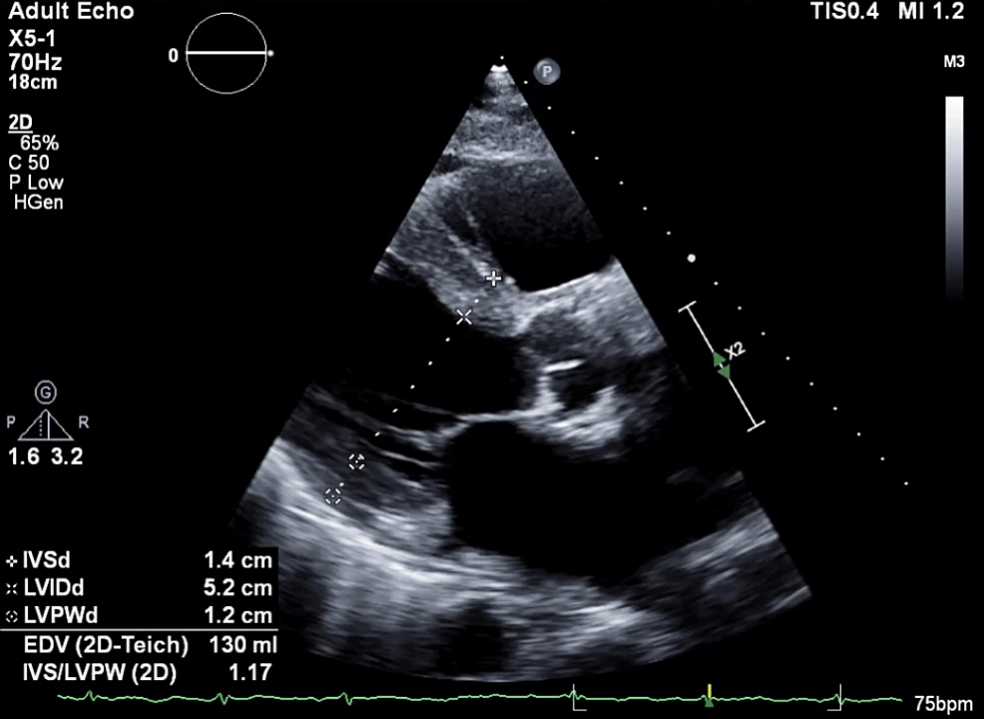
A parasternal long-axis view of an echocardiogram indicated moderate concentric left ventricular hypertrophy with a posterior wall thickness of 12 mm and a thickened interventricular septum measuring 14 mm IVSd: interventricular septal end diastole thickness; LVIDd: left ventricular internal diameter end diastole; LVPWd: left ventricular posterior wall end diastole thickness; EDV: end-diastolic volume; IVS/LVPW: the ratio of interventricular septum thickness to left ventricle posterior wall thickness
